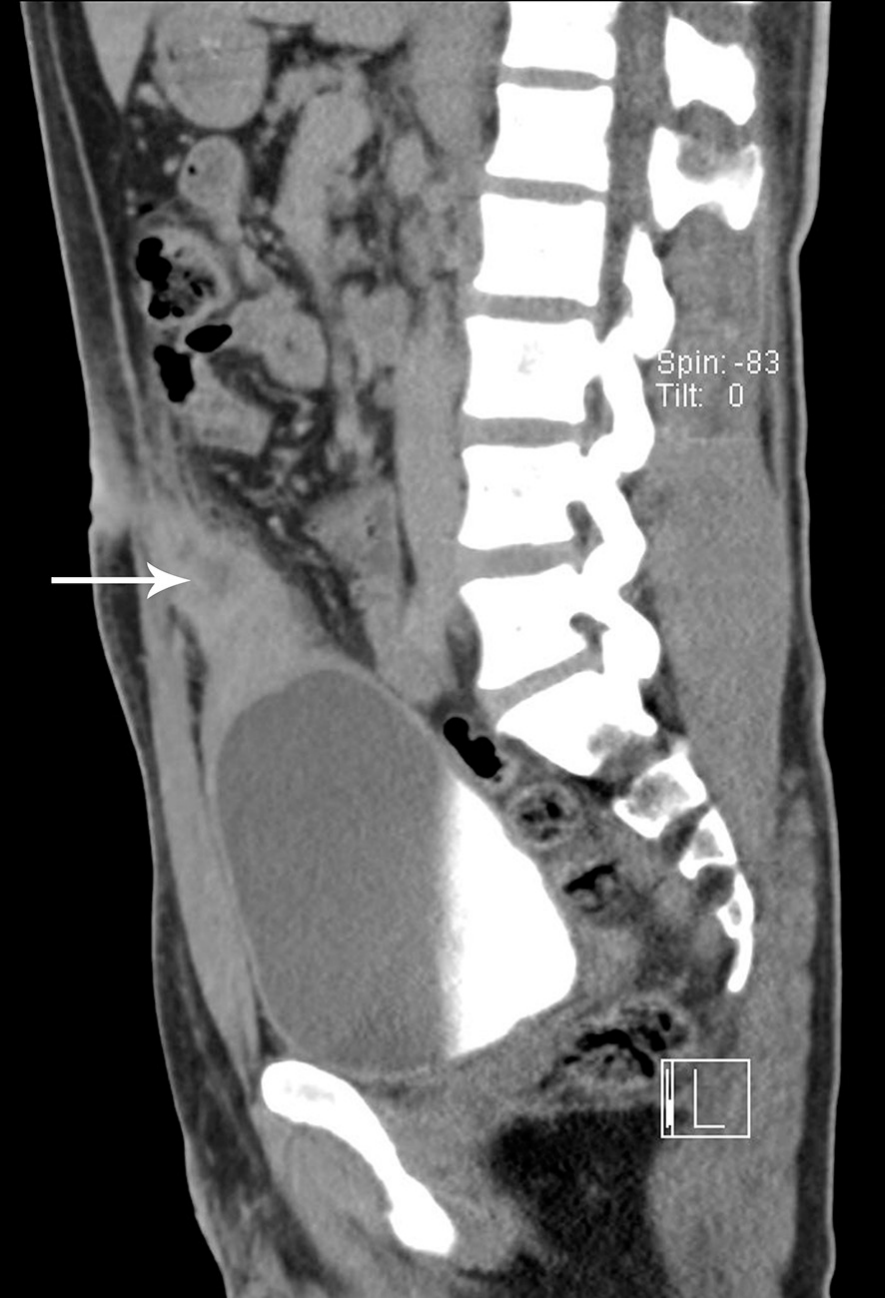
Sagittal CT image showing hypodense mass, anterior and superior to the vesical dome extending to the umbilicus. The presence of liquid collection adjacent to the umbilicus (arrow) is significant.
